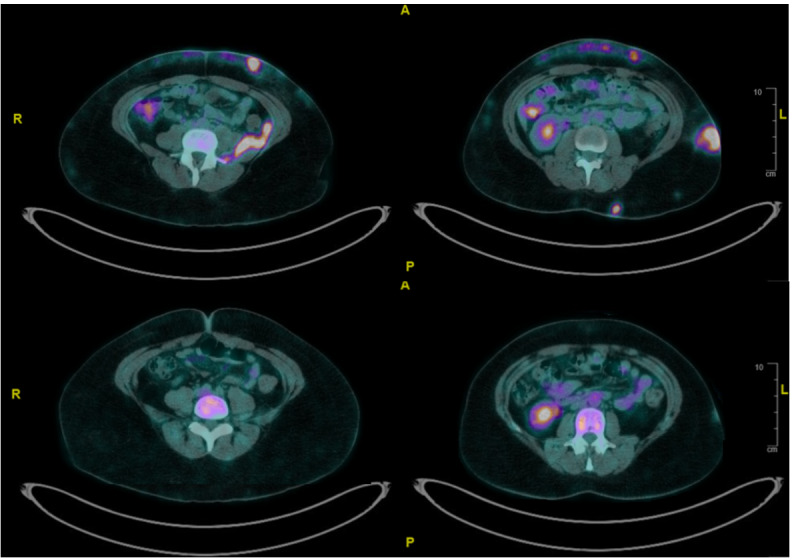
PET scan of abdomen of first case with disseminated SPTCL (top) before treatment: FDG avid sub-cutaneous nodules, lymphadenopathy and retroperitoneal soft tissue thickening. (bottom) 4 months into treatment: Previously noted areas of sub-cutaneous thickening and nodularities have resolved, especially in the anterior and left lateral abdominal walls.
